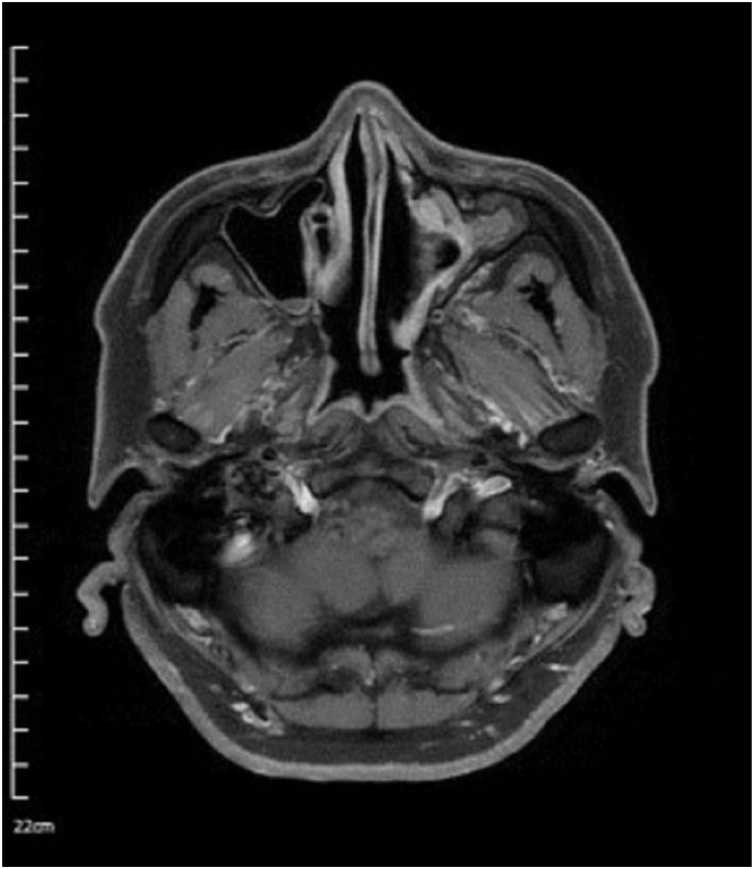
Radiology findings after the wide local excision (3rd surgery). The paranasal sinus magnetic resonance imaging axial T1-weight contrast-enhanced view revealed a thickened contrast-enhanced mass along the mucosa of the maxillary sinus and nasolacrimal duct.
